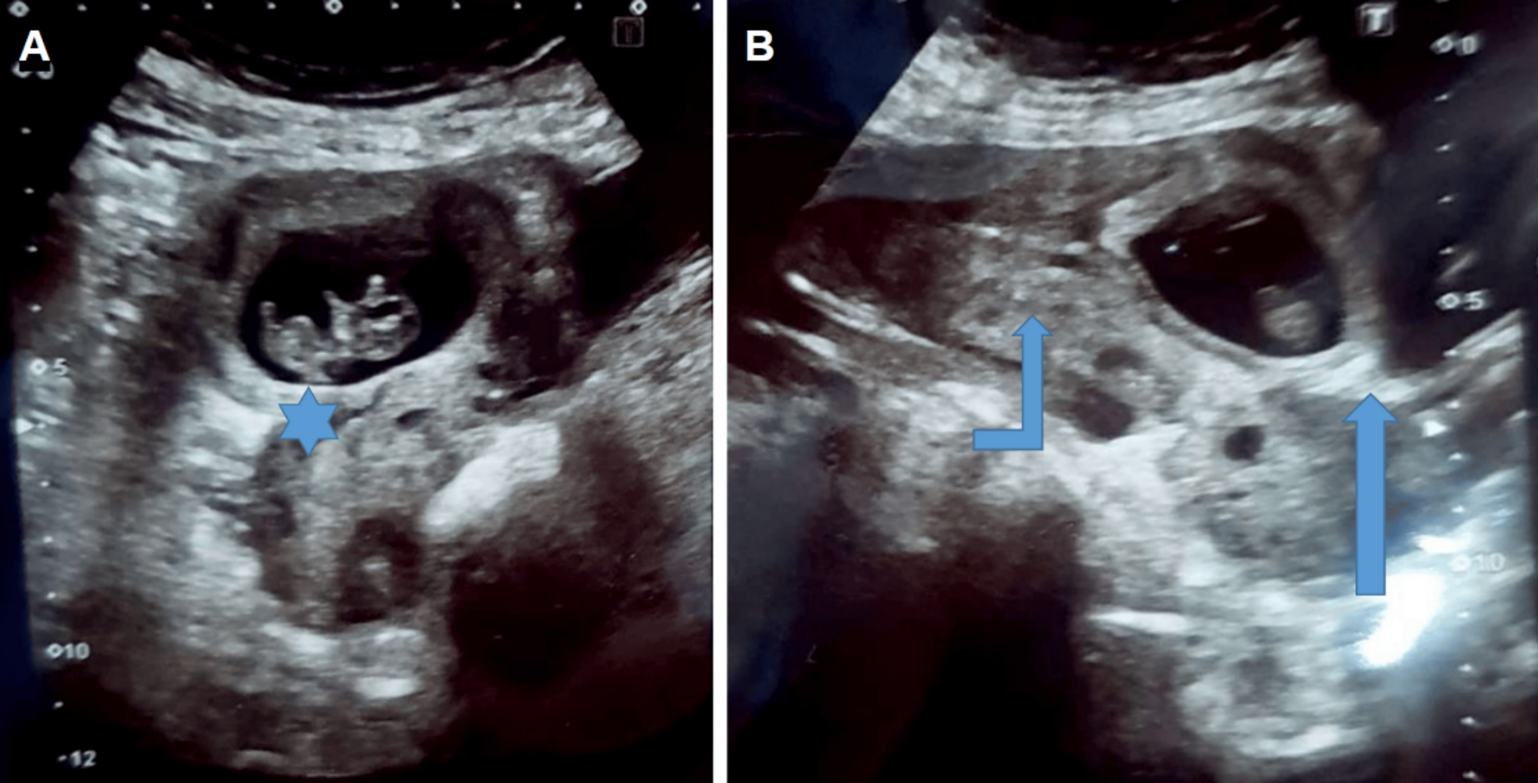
Transabdominal ultrasound pelvis A, B: Empty uterine cavity and cervical canal (curved arrow), gestational sac containing viable fetus (asterisk) in anterior lower uterine segment with no intervening myometrial tissue between bladder wall and uterus (straight arrow).
